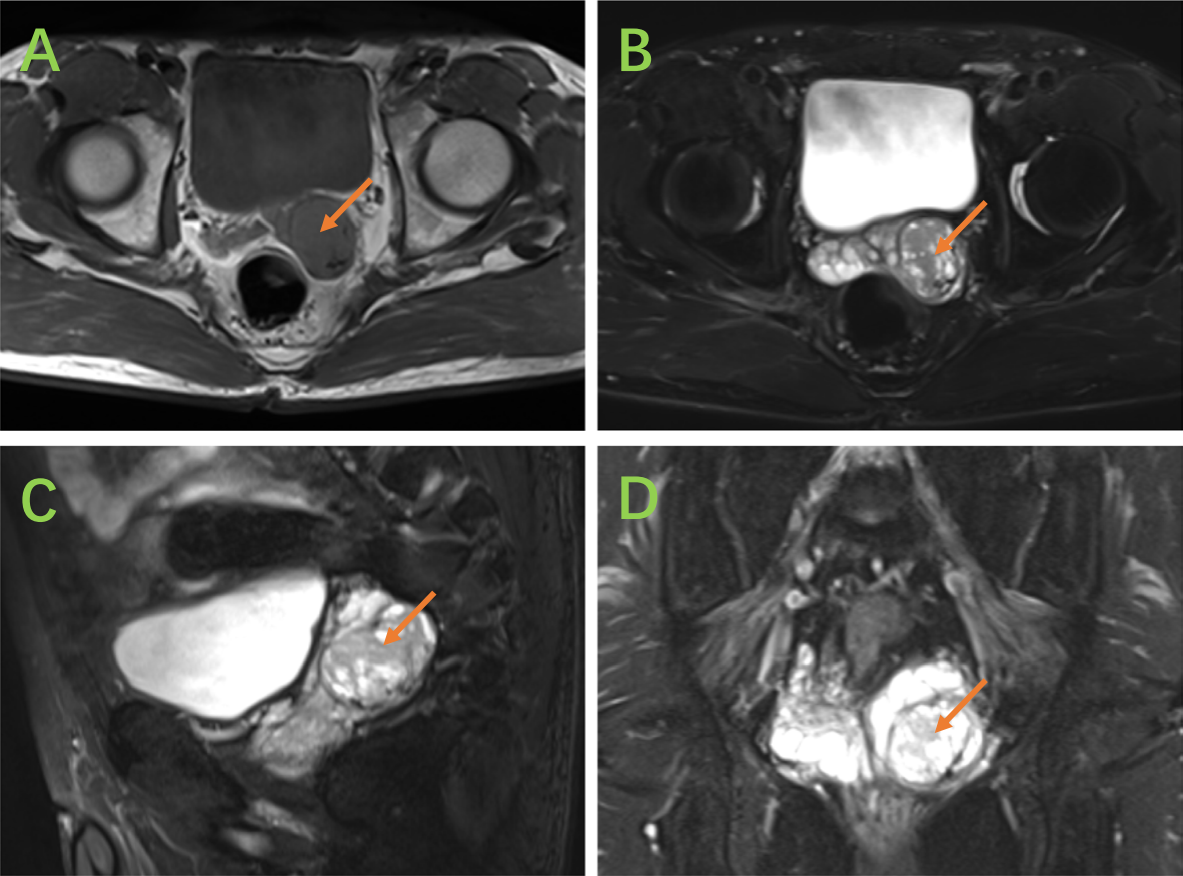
A Axial magnetic resonance imaging (MRI) in the T1 phase; B Axial MR image in the T2 phase; C Sagittal MR image in the T2 phase; D Coronal MR image in the T2 phase
